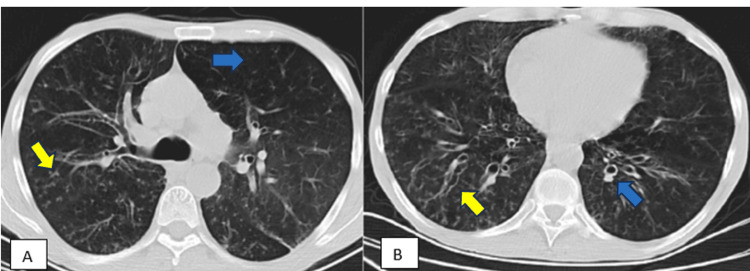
Thoracic CT showing widespread central and peripheral nodular infiltrations, moderate bronchiectasis, air-trapping areas, and bronchial wall thickening in both lungs, predominantly in the middle and lower zones. (A) CT of the thorax shows widespread peripheral and central nodular infiltrations (yellow arrow) and air-trapping areas (blue arrow). (B) CT of the thorax shows moderate bronchiectasis (yellow arrow) and bronchial wall thickening in both lungs (blue arrow), predominantly in the middle and lower zones.
